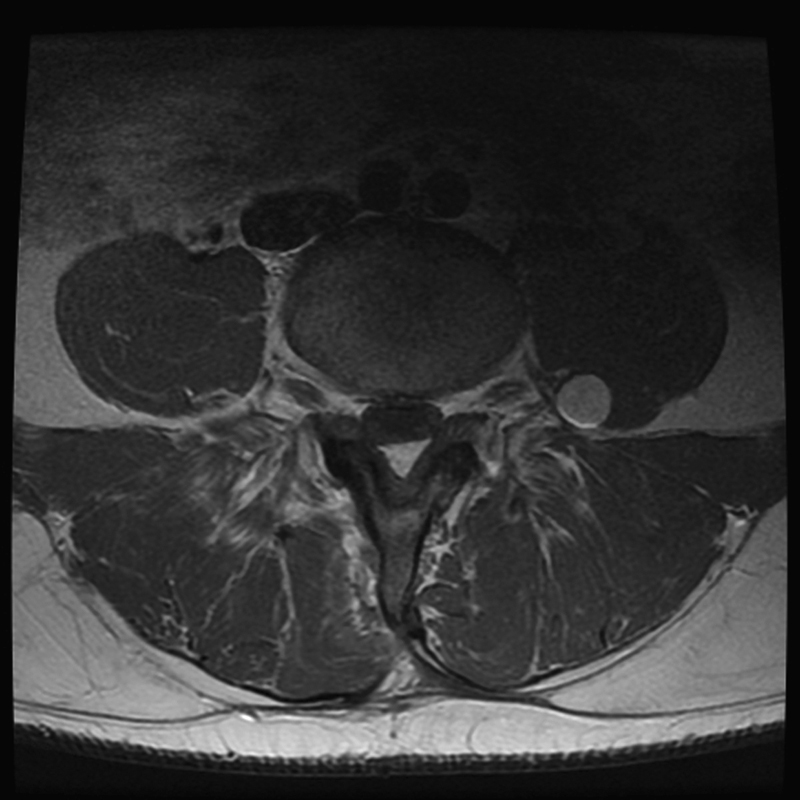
Preoperative axial magnetic resonance imaging with contrast demonstrating enhancing mass in the left psoas muscle.
